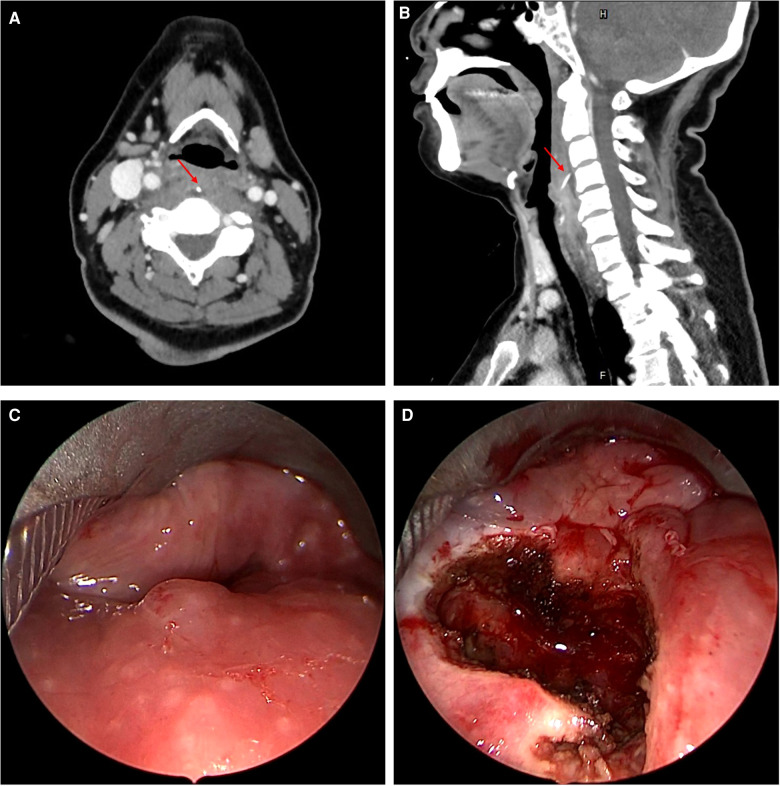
(A, B) Axial and sagittal CT images of patient on initial presentation; (C) laryngoscopy view of the posterior wall of the pharyngeal cavity before incision during the first surgery; (D) laryngoscopy view after incision of the pharyngeal mucosa during the first surgery. CT = computed tomography.
